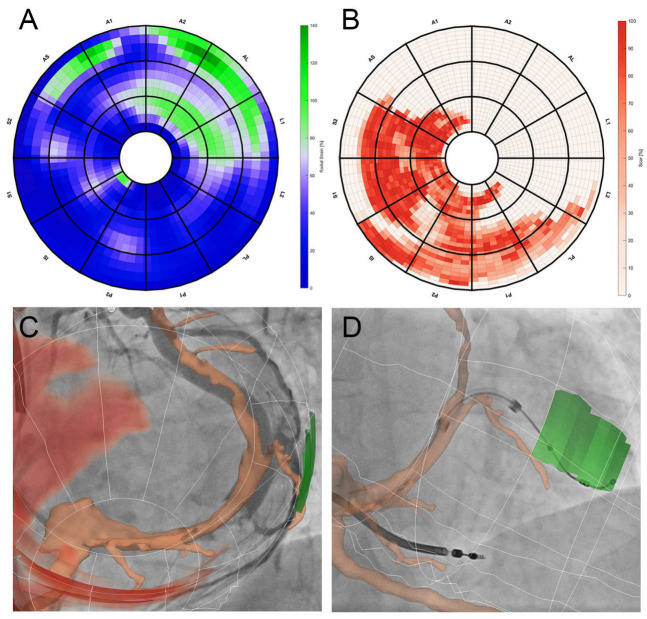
CT-based live-guided CRT placement. The maximum radial strain at the end of the cardiac cycle is located in the anterolateral segment (AL) of the left ventricle as is shown on the bulls-eye plot (panel (A)), and the scar is located in the septal-posterolateral (S2-PL) part of the myocardium (panel (B)). These areas are combined with the CT venogram to create a 3D rendering of the bulls-eye plot (with the optimal target in green and scar in red) and the coronary veins, which are projected on top of the angiography image during CRT implantation (panel (C)). The final lead position is within the target area, outside of the scar (which is omitted for clarity in this recording) (panel (D)).
